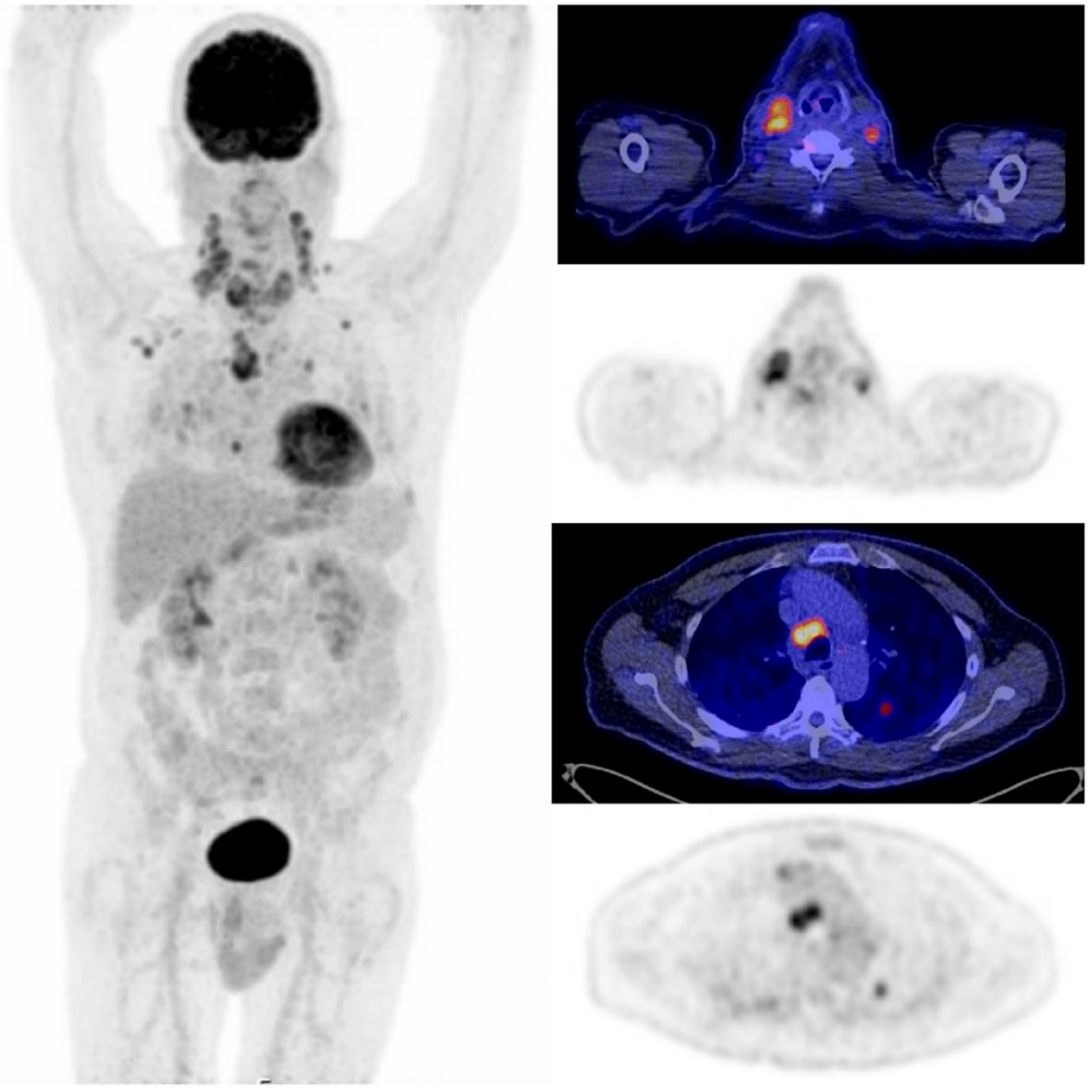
^18^F-FDG PET/CT of a patient with extensive bilateral lymph node metastases in the neck and distant metastases in lungs
